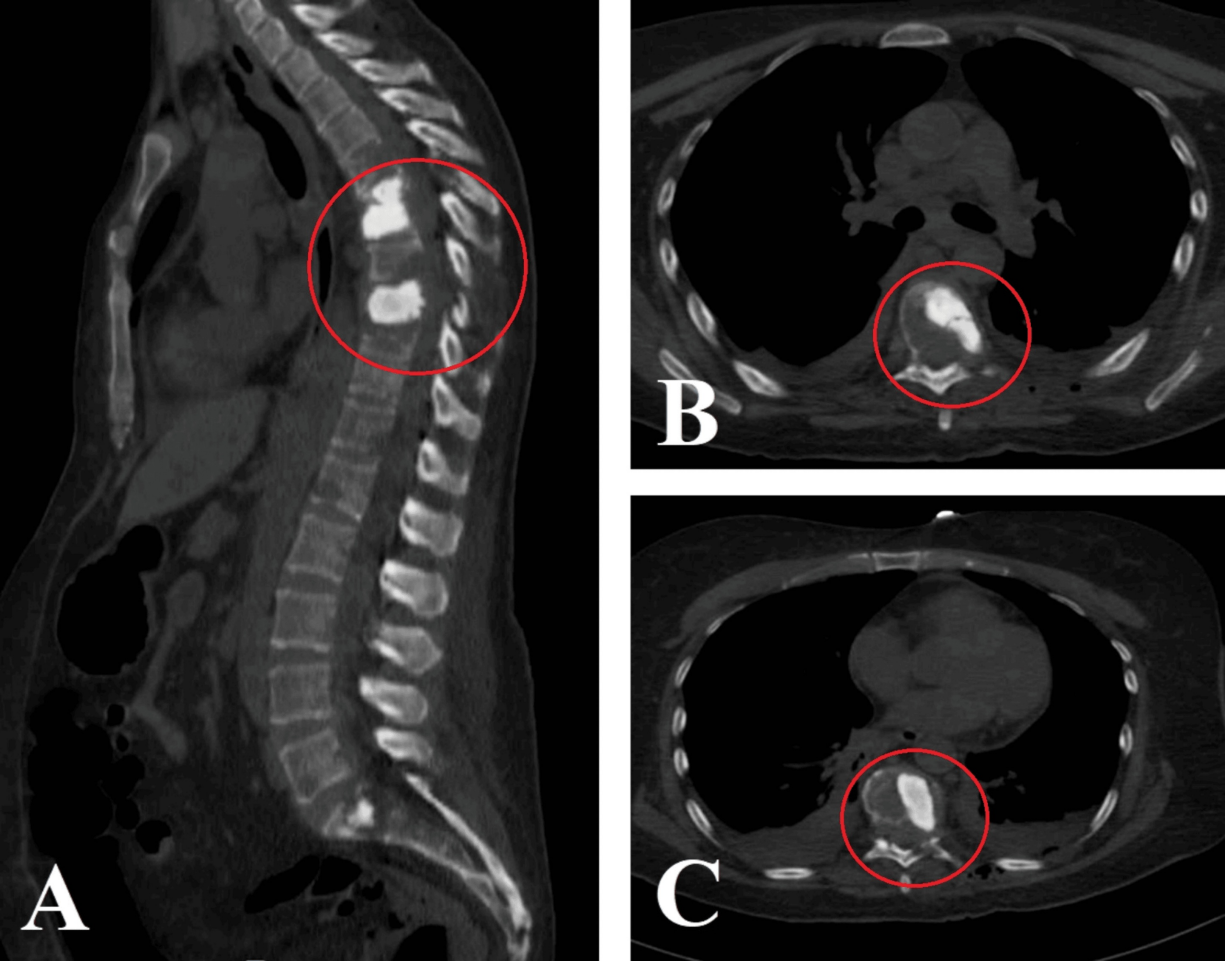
Sagittal (A) and axial (B, C) CT scans of the thoracic spine show vertebral augmentation of the T6 and T9 vertebral bodies with methylmethacrylate, corpectomy of the posterior walls of these vertebrae, and an increase in the space of the medullary canal.
